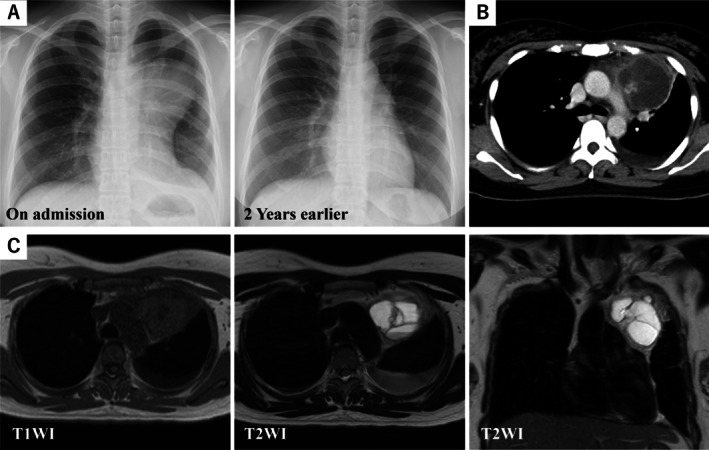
Radiological findings of the anterior mediastinal mass. (A) Chest radiograph revealing a new anterior mediastinal mass that was not present on imaging 2 years earlier. (B) Contrast‐enhanced CT showing a 5.9 × 6.1 × 6.8 cm anterior mediastinal mass, primarily composed of multilocular cystic components with minimal solid portions. Partial enhancement is seen along the internal septa. Left‐sided pleural effusion and pericardial fluid are also present. (C) MRI demonstrating low signal intensity on T1‐weighted images and high signal intensity on T2‐weighted images within the cysts. In contrast, the cyst walls appeared hypointense on both T1 and T2‐weighted images.
